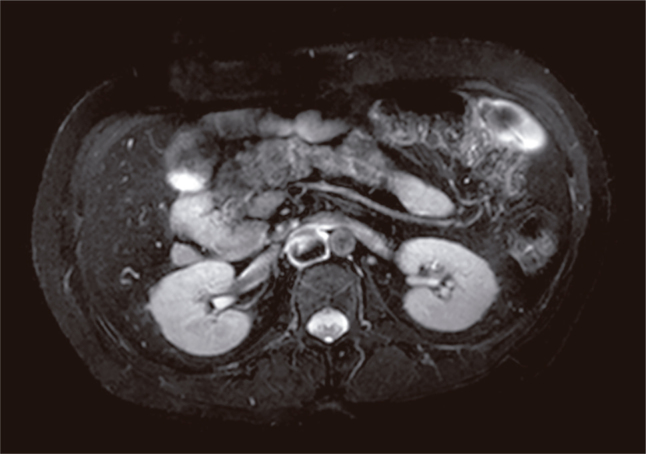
Abdominal MRI and cholangiopancreatography MRI showing agenesis of dorsal pancreas with increased dimensions of the pancreas head.
